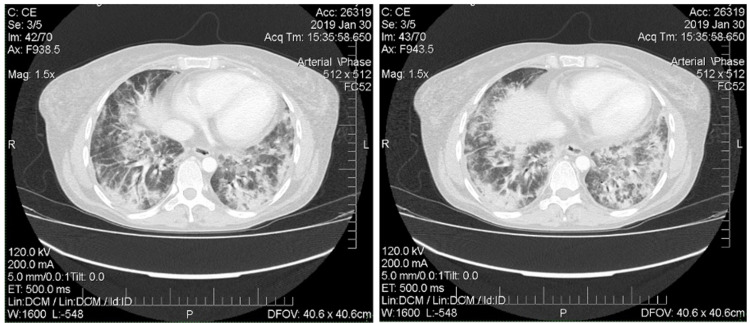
Multislice computed tomography of the chest (January 2019). Horizontal tomography slices on different levels of thorax demonstrate confluent shadows and foci of lung tissue infiltrate from 3 to 45 mm in diameter without clear contours.
